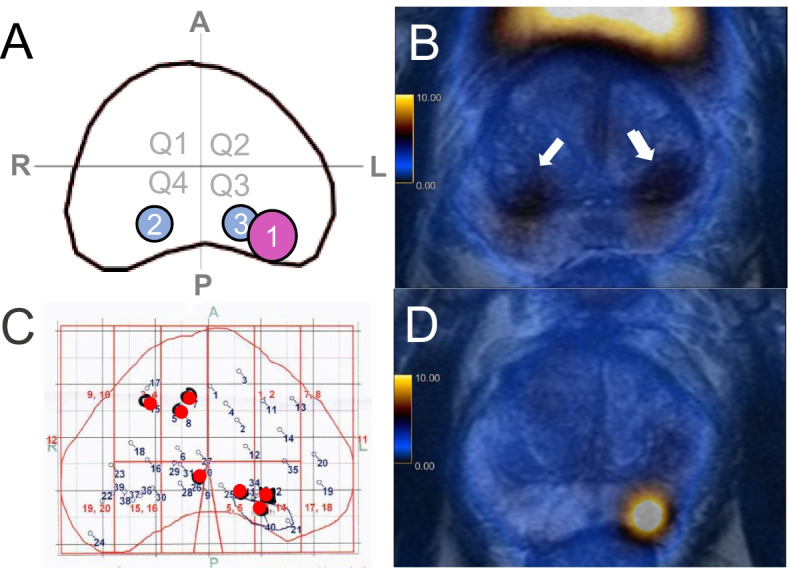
Example of the method used for lesion localization. A Readout of PSMA PET/MRI by one of the readers with the prostate gland divided in 4 quadrants (Q1: anterior right; Q2: anterior left; Q3: posterior left and Q4: posterior right). The reader delineated three areas of ^68^Ga-PSMA-11 uptake and labeled area 1 as suspicious for malignancy and areas 2 and 3 as benign/physiological. B
